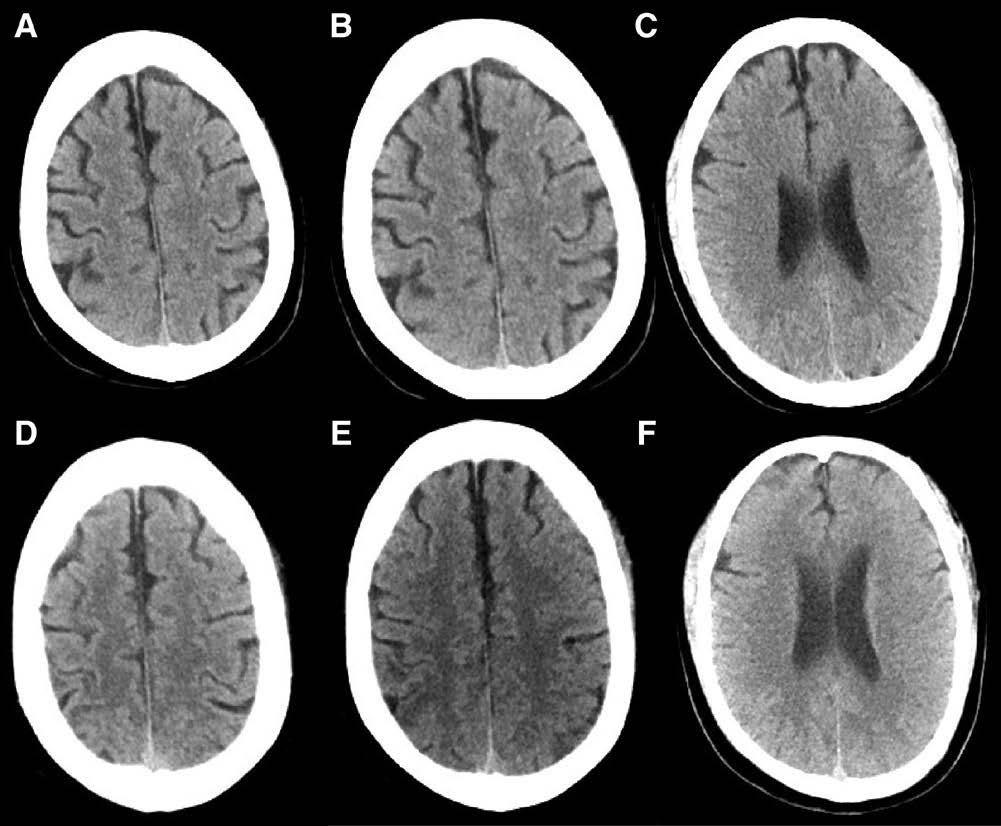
First cranial CT scan of the patient on the 7th day of admission (A–C): no obvious abnormalities were found; second cranial CT scan on the 11th day of admission (D–F): local density reduction in right parietal lobes may be seen indistinctly. CT = computed tomography.
